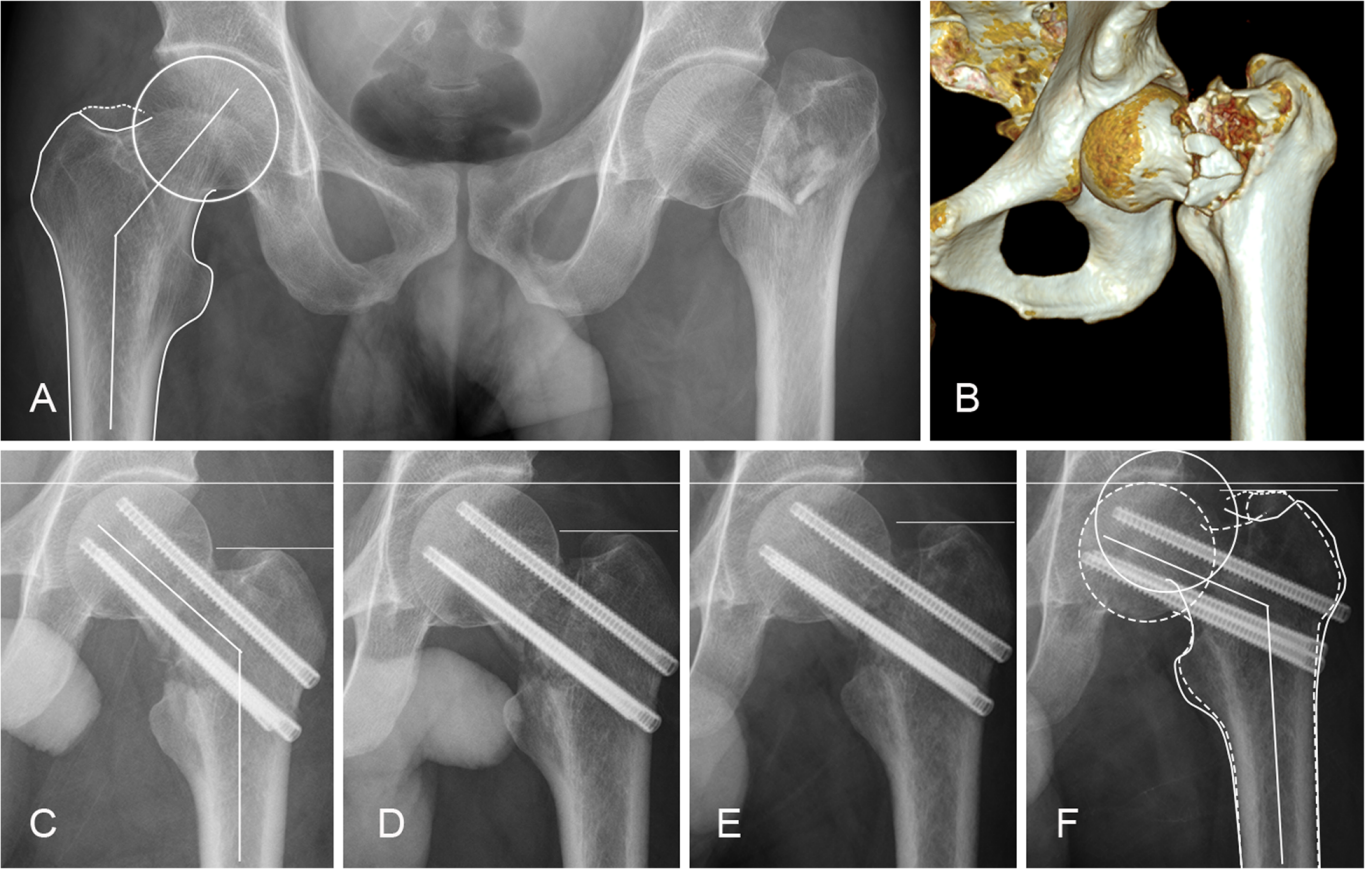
FTHCS fixation for FNF in a 40-year-old complicated by femoral neck shortening. The pelvic AP radiograph (a) and CT scan 3-D reconstruction images (b) showed the right hip with a comminuted femoral neck fracture. a The uninjured hip was outlined and the angle between the axis of the head and shaft (neck-shaft angle) was labeled. Immediate postoperative radiographs (c) showed near anatomical reduction with corrected neck-shaft angle. One-month (d) and 3-month (e) postoperative radiographs showed stability of the fixation. Unfortunately, 2-year radiographs (f) demonstrated fracture union with significant femoral neck shortening and varus displacement. The outline of the uninjured hip (solid line) overlapped on the fracture side (dotted line) is provided for comparison. From (c) to (f), two horizontal lines were drawn on each radiograph, one from the top of the femoral head and another from the tip of the greater trochanter. The difference in the measurement between these two horizontal lines revealed the amount of head collapse
